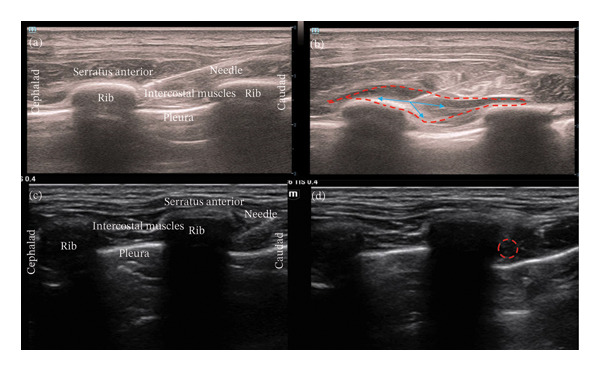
Ultrasound images of MINB preinjection (Figure (a) and postinjection (Figure (b)); ultrasound images of UINB preinjection (Figure (c)) and postinjection (Figure (d)). Notes: Blue arrows indicate the direction of local anesthetic spread; red dashed line delineates the spread area. Abbreviations: MINB, ultrasound‐guided modified intercostal nerve block; UINB, ultrasound‐guided intercostal nerve block.
